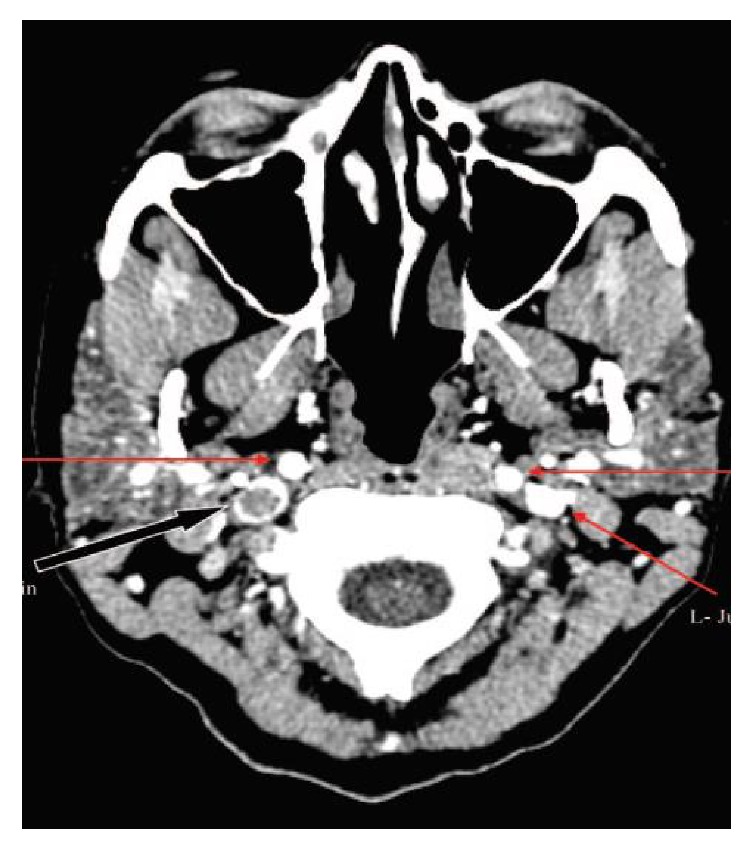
Computed tomographic venogram shows direct visualization of thrombus within the right internal jugular vein in another patient, marked by black arrow [6]. Red arrows mark normal flow voids. Reprinted from [6].
